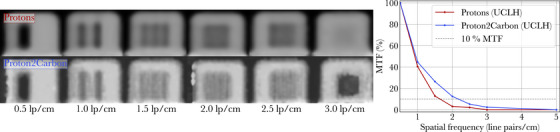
Ion radiographs of line pairs modules and associated modulation transfer functions (MTFs). The line pair modules were scanned at a different institution as the training data used for the Proton2Carbon model.
